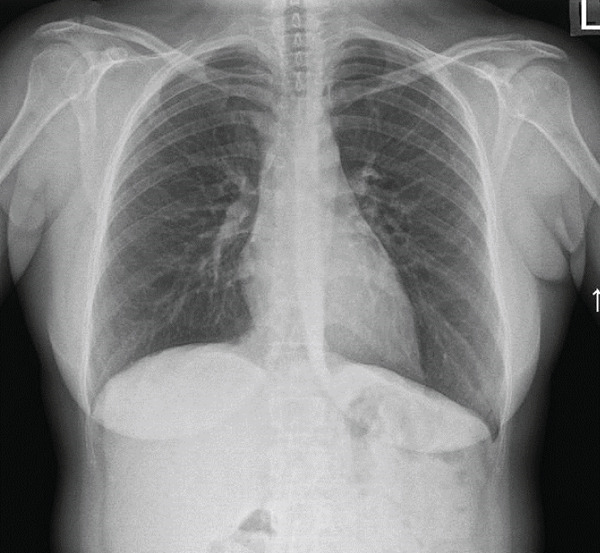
Follow‐up chest radiograph after treatment (September 2024).
